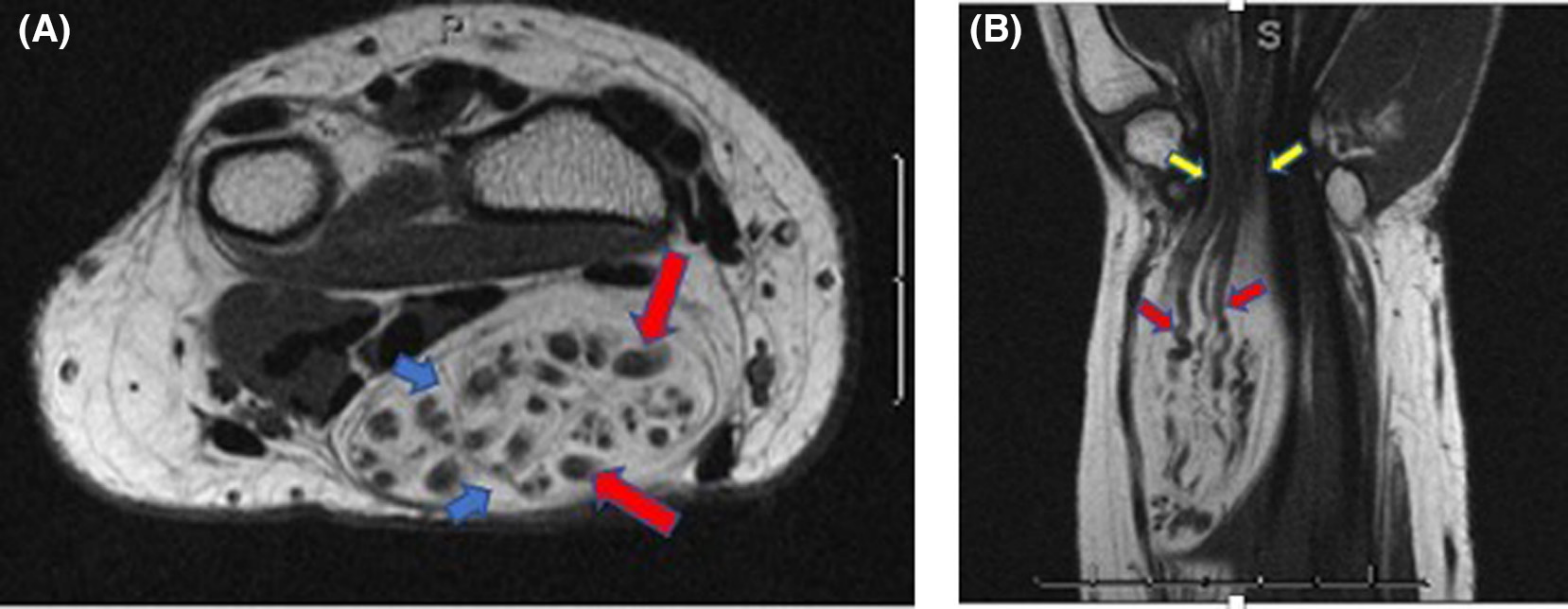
Magnetic resonance imaging of the fibrolipomatous hamartoma in T1‐weighted images showing typical findings. (A), Axial image demonstrating marked enlargement of the median nerve. Low‐intensity structures (nerve fascicles) (red arrows) are surrounded by high signal intensity (fat) (blue arrows) resembling a “co‐axial cable‐like appearance,” for the nerve in the distal forearm. (B), Coronal image demonstrating the median nerve fascicles (red arrows) inside the same large mass of the distal forearm, as serpiginous low‐intensity structures surrounded by fat, with “spaghetti‐like appearance.” Note the marked constriction of the mass inside the carpal tunnel (yellow arrows).
